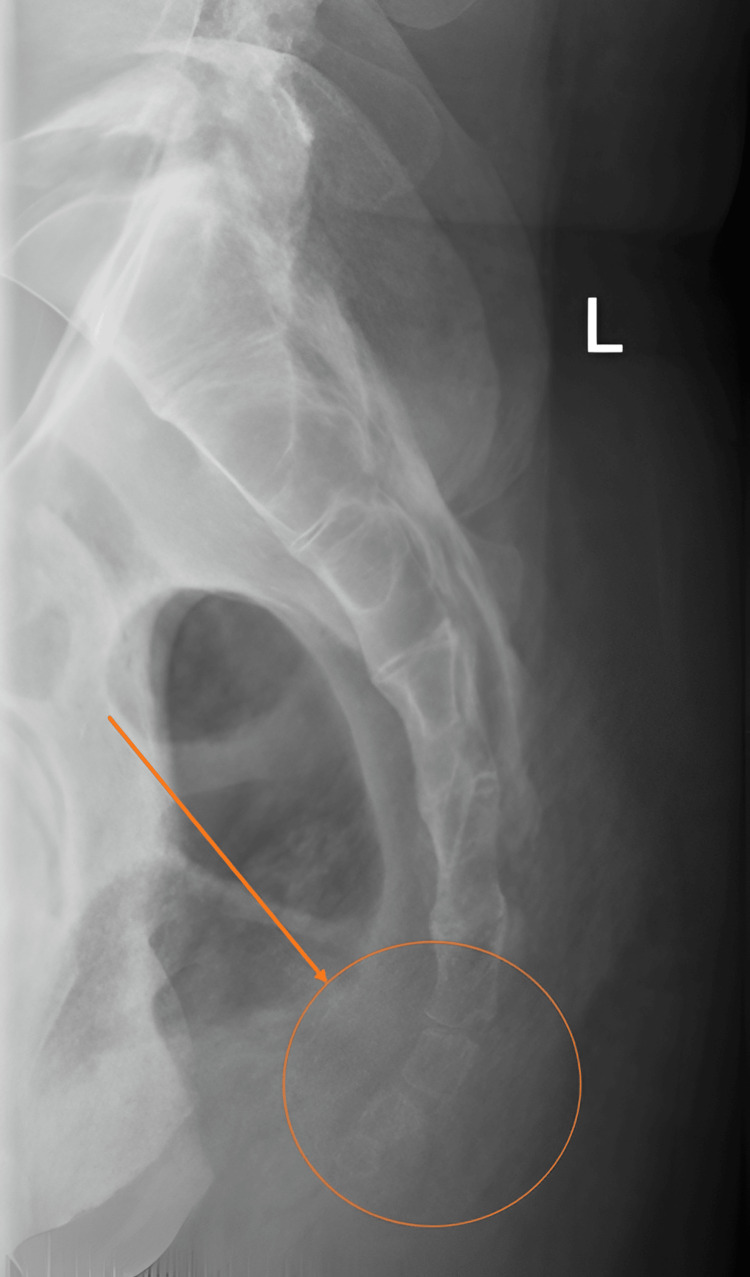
Lateral radiograph of the sacrum and coccyx showing the normal five sacral and four coccygeal segments
